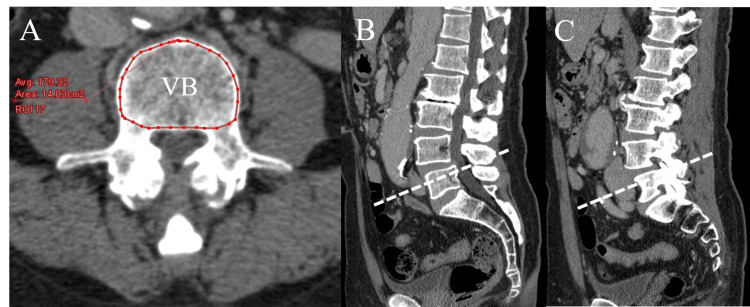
Measurement of the vertebral body cross-sectional area (A) Axial reconstructed CT images. Measurements of the cross-sectional area of the vertebral body (VB) are shown. (B and C) Sagittal reconstructed CT images showing the level from which measurements are taken: at the middle level of the L4 pedicle (white dotted line).
